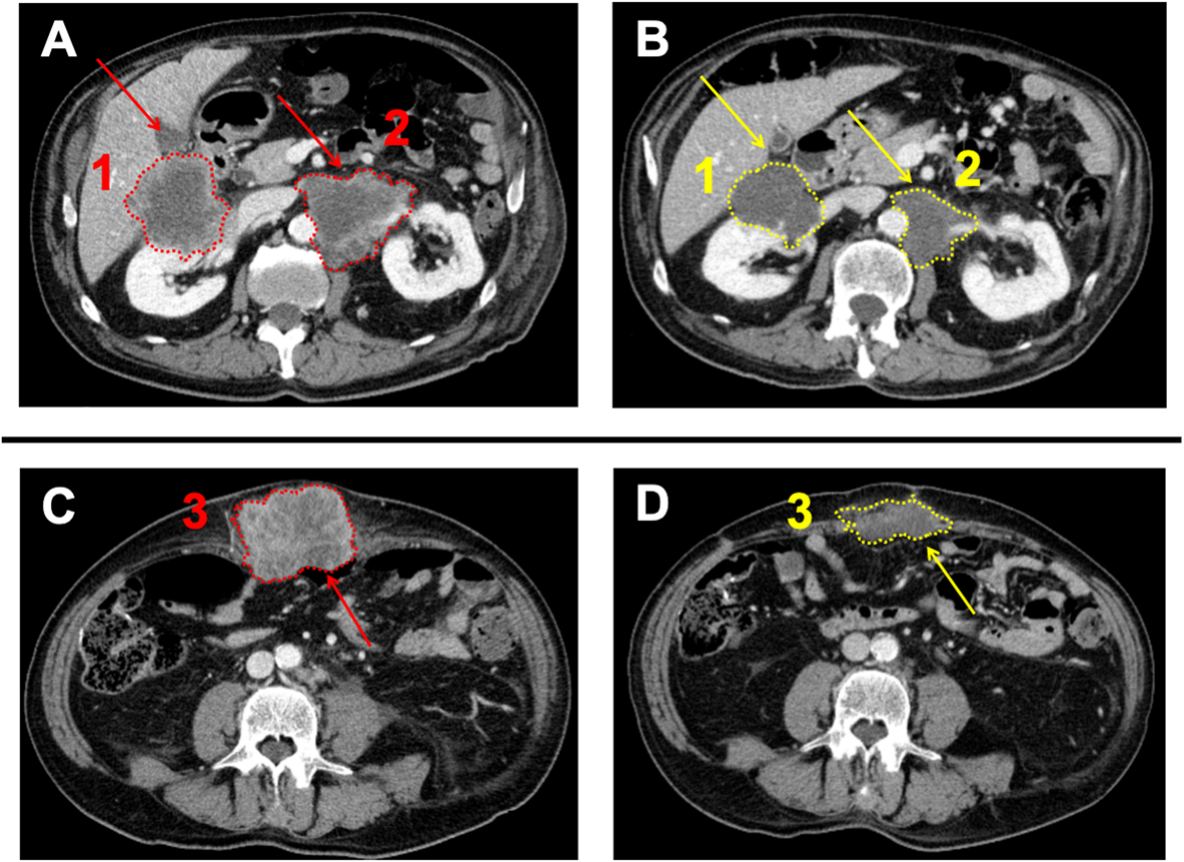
a-c Baseline CT scan images before ICI start, June 2015 (red arrows and dashed perimetral lines). Lesion A1: maximum diameter 77 mm (mm), estimated volume 1240 × 10^3^ cubic millimetres (mm^3^). Lesion A2: maximum diameter 76 mm, estimated volume 935 × 10^3^ mm^3^. Lesion C3: maximum diameter 96 mm, estimated volume 1191 × 10 [3] mm^3^. b-d Best response CT scan images, Sep 2015 (yellow arrows and dashed perimetral lines). Lesion B1: maximum diameter 48 mm, estimated volume 422 × 10^3^ mm^3^. Lesion B2: maximum diameter 42 mm, estimated volume 412 × 10 [3] mm^3^. Lesion D3: maximum diameter 79 mm, estimated volume 216 × 10^3^ mm^3^
